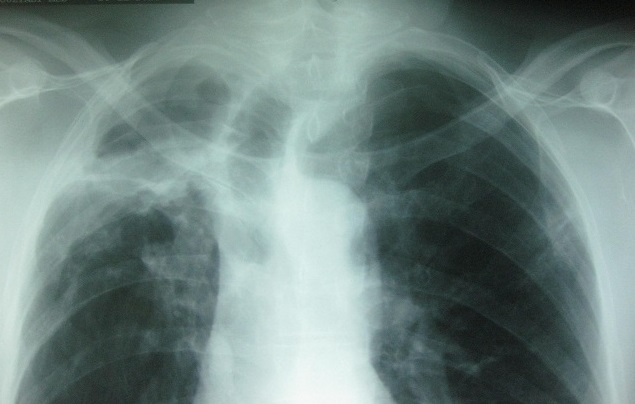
Radiographie pulmonaire montrant une opacité au niveau du lobe supérieur droit avec infiltrats parenchymateux adjacents
